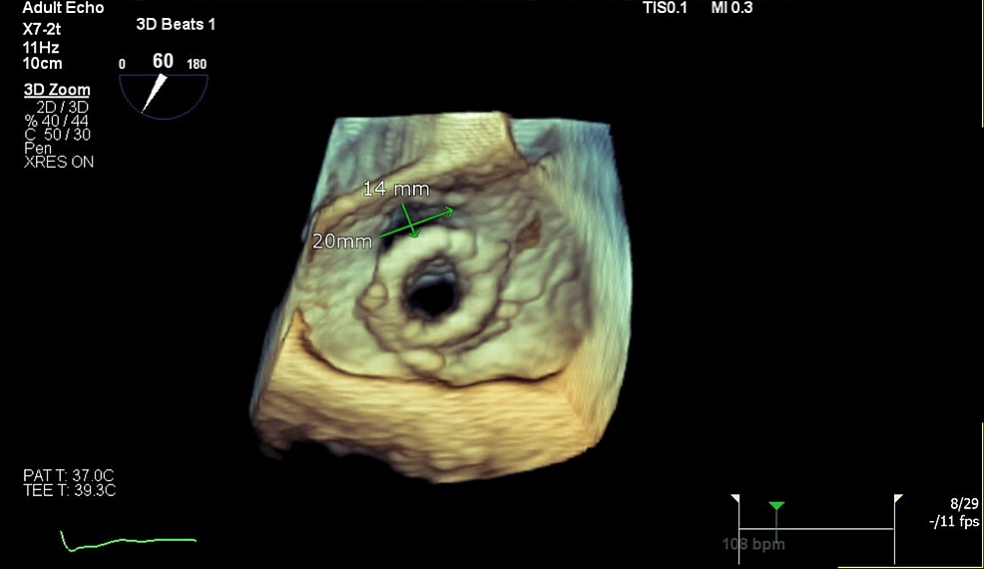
The 3D TEE shows the PVL (20 x 14 mm) defect around the bioprosthetic valve causing severe TR. TEE: Transesophageal echocardiography, PVL: Paravalvular leak, TR: Tricuspid regurgitation
